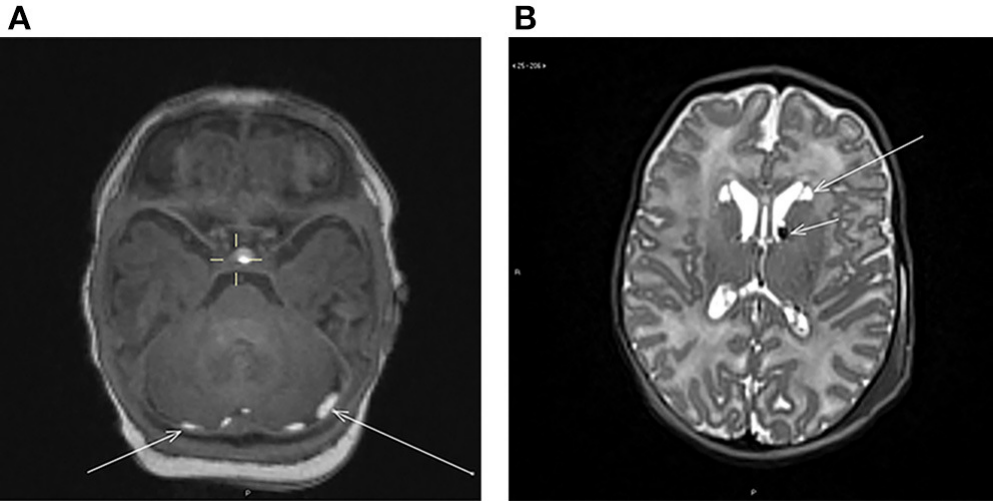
(A) Axial T1-weighted image showing bilateral subdural hemorrhages (arrows) in the posterior fossa behind the cerebellum. (B) Axial T2-weighted image showing bilateral cysts at the head of nucleus caudatus (long arrow) and bilateral hemorrhages at the caudothalamic groove (short arrow).
